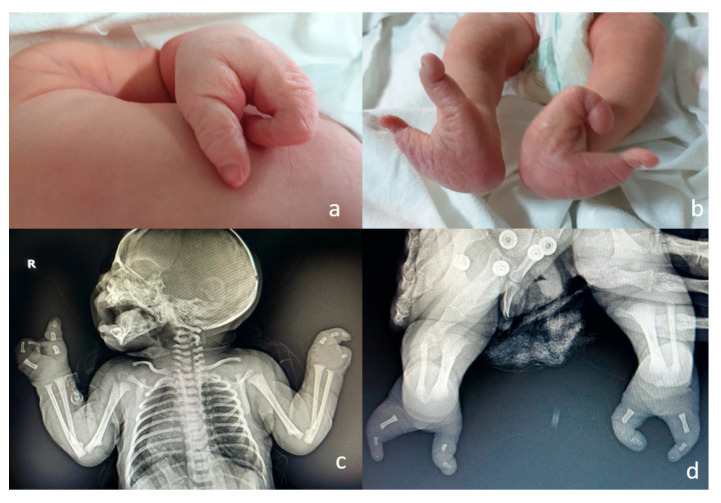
Ectrodactyly—this case was missed in the prenatal period. A single third-trimester scan was performed, at 36 weeks of amenorrhea. (a,b)—postnatal aspect: split hand and split foot malformation; the absence/defect of the central fingers and toes may be appreciated, giving the “lobster claw” appearance. (c,d)—postnatal CT, confirming the clinical aspect.
